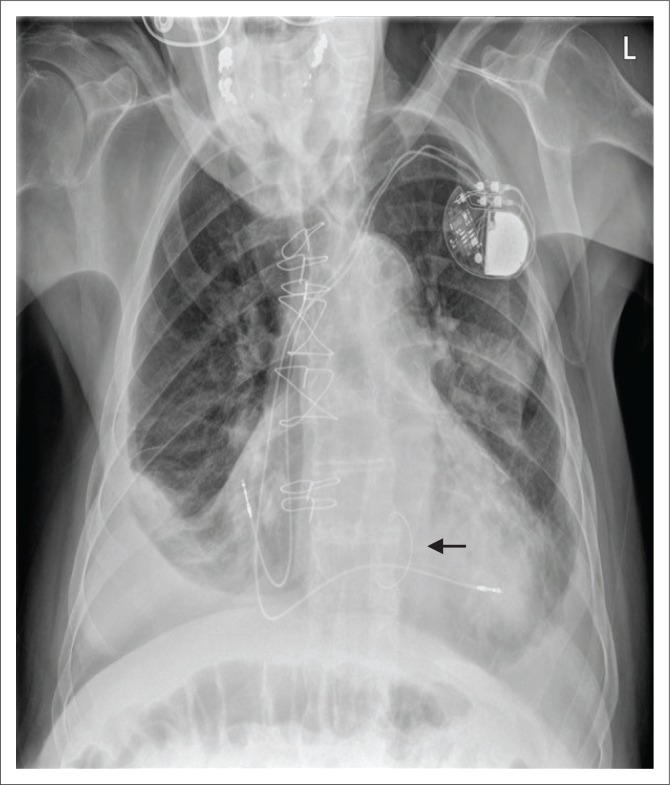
Chest radiograph showing a mitral annuloplasty C ring (arrow). Note the dual-lead pacemaker with accurately placed right atrial and right ventricular leads.
